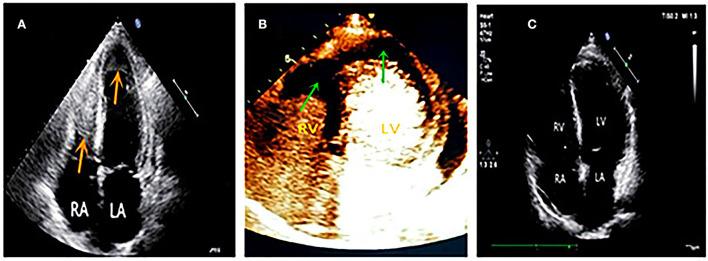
(A) Four chamber view showed masses in both of the ventricular apex (red arrows). (B) Contrast echocardiography showed no perfusion in both apical masses (green arrows), which suggested thrombi. (C) Follow-up at six months later, both ventricular apical thrombi were disappeared.
